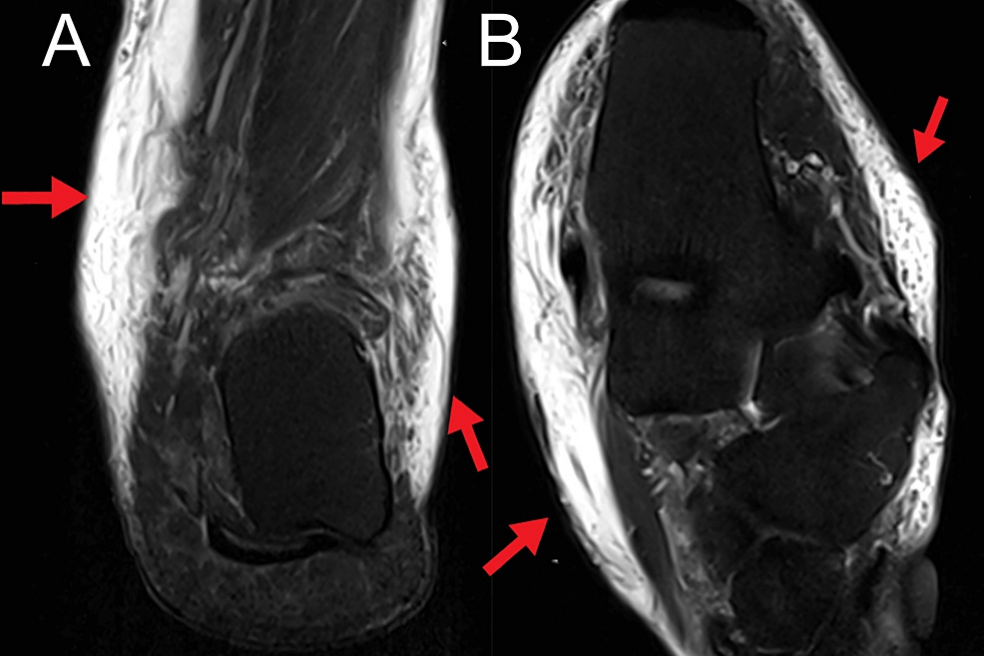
Left ankle magnetic resonance image. (A) On the coronal image, diffuse subcutaneous soft tissue edema around the ankle is noted (arrows). (B) On the axial image, subcutaneous soft tissue edema around the ankle is also noted (arrows). All findings are suggestive of cellulitis.
